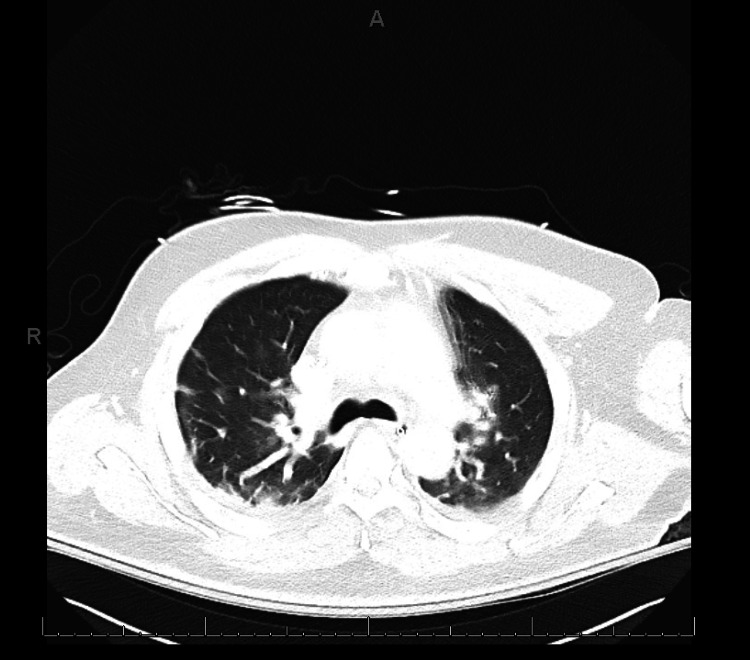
Computed tomography of chest
